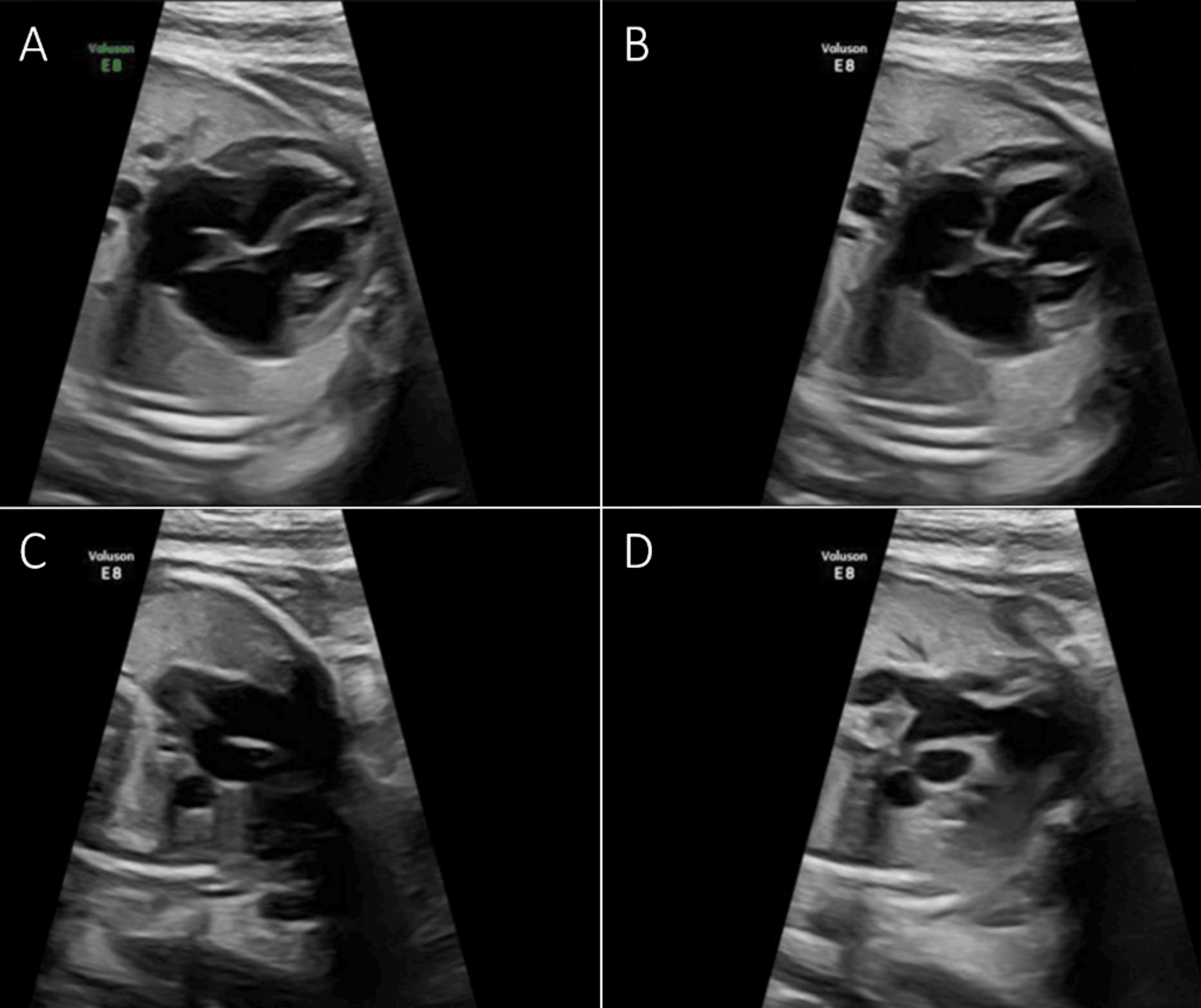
Fetal echocardiography performed at 34 weeks of gestation Fetal echocardiography was performed, demonstrating normal cardiac anatomy and function throughout the cardiac cycle. Images A and C correspond to diastole, showing ventricular relaxation with open atrioventricular valves (A: four-chamber view; C: left ventricular outflow tract). Images B and D correspond to systole, with ventricular contraction and closed atrioventricular valves (B), and aortic valve opening with forward flow through the LVOT (D). No structural abnormalities or functional impairment were identified.
